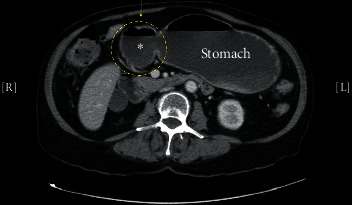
Initial abdomen computed tomography scan showing nonspecific fluid distension of the stomach with possible diverticulum (circle).
